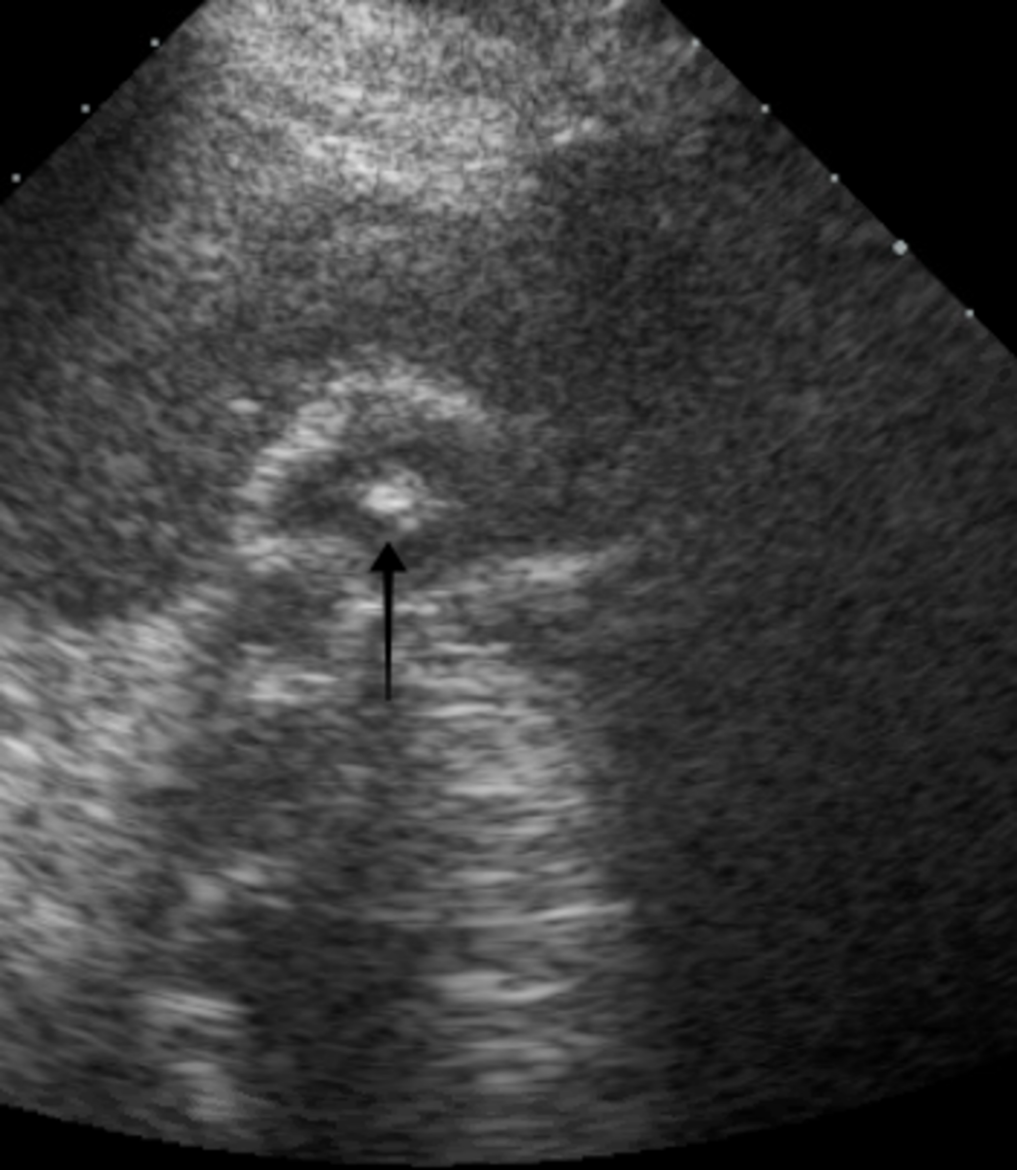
Ultrasonography of the abdomen revealing cholelithiasis
